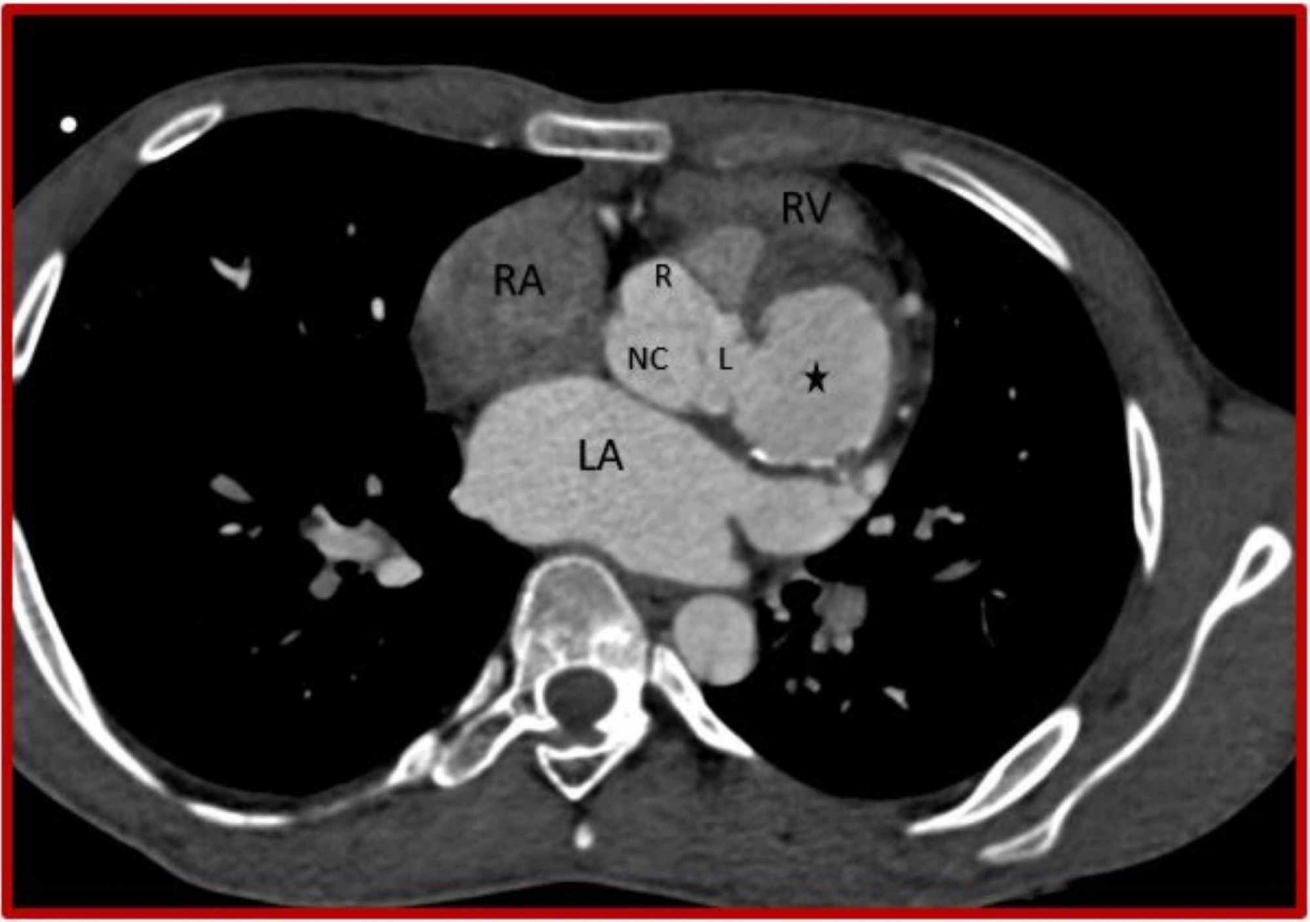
Cardiac CT (Axial view) demonstrates left sinus of Valsalva aneurysm (asterisk) with peripheral mural calcifications. CT: Computed tomography; RV: Right ventricle; RA: Right atrium; LA: Left atrium; R: Right coronary sinus; L: Left coronary sinus; NC: Non-coronary sinus.
